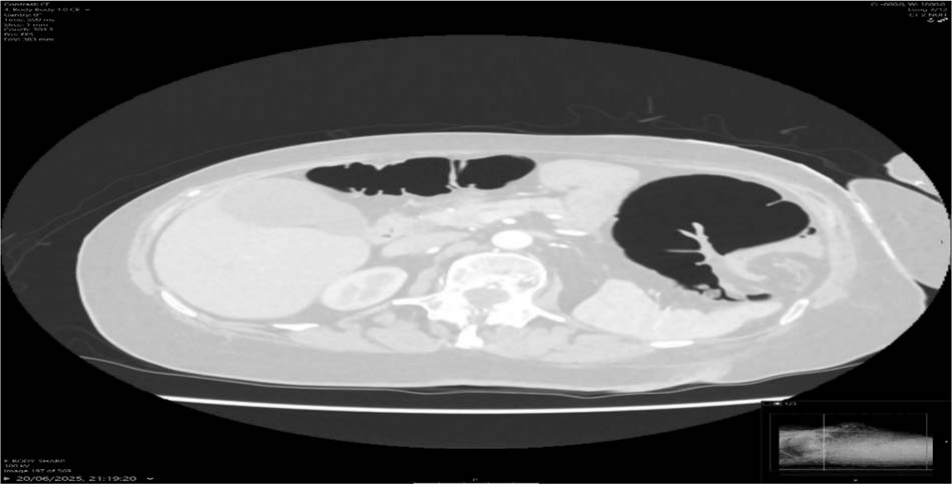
Axial CT lung window showing no evidence of perforation.
